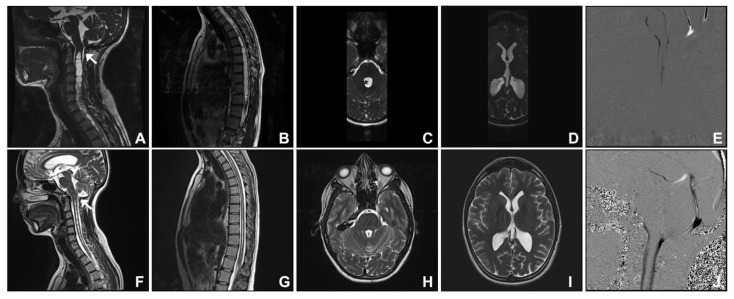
Chiari mimic: post-traumatic cranio-cervical junction arachnoiditis. A 26-year-old woman presented with a history of premature birth and a prolonged period in the neonatal intensive care unit. Investigations revealed what appeared to be a Chiari I malformation with a holocord syringomyelia (A,B). Additional magnetic resonance imaging (MRI) features include septa at the cervico-medullary junction consistent with cranio-cervical junction arachnoiditis ((A); white arrow), enlarged fourth ((C); * for enlarged fourth ventricle) and lateral ventricles (D), and a lack of dorsal cerebrospinal fluid (CSF) flow evident on phase contrast CSF flow studies (E). Posterior fossa decompression and shunting of the fourth ventricle to the spinal subarachnoid space was undertaken ((F); white arrow for shunt). At follow-up, there was full resolution of the symptoms in addition to reduction in syrinx size (G) and the fourth and lateral ventricles’ size (H,I), and established dorsal CSF flow on phase contrast CSF flow studies (J).
